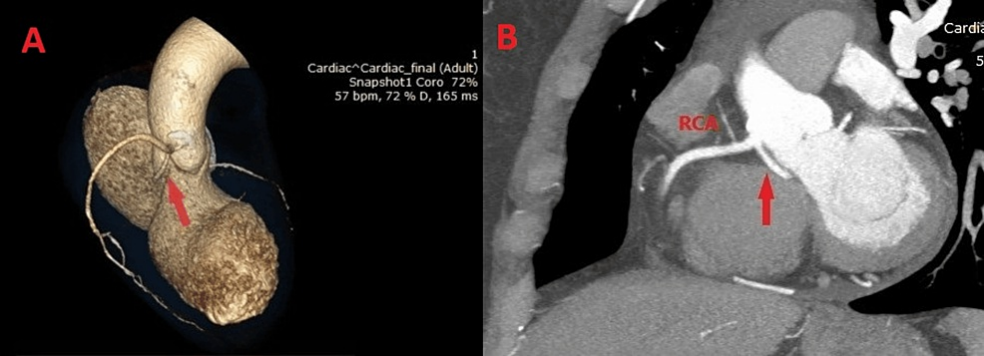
Case 2 A) Three-dimensional CCTA image showing LM (red arrow) originating from the right coronary sinus with retro-aortic course. B) Two-dimensional CCTA image showing LM (red arrow) originating from the right coronary sinus with retro-aortic course. CCTA: Coronary CT Angiogram; LM: Left Main
